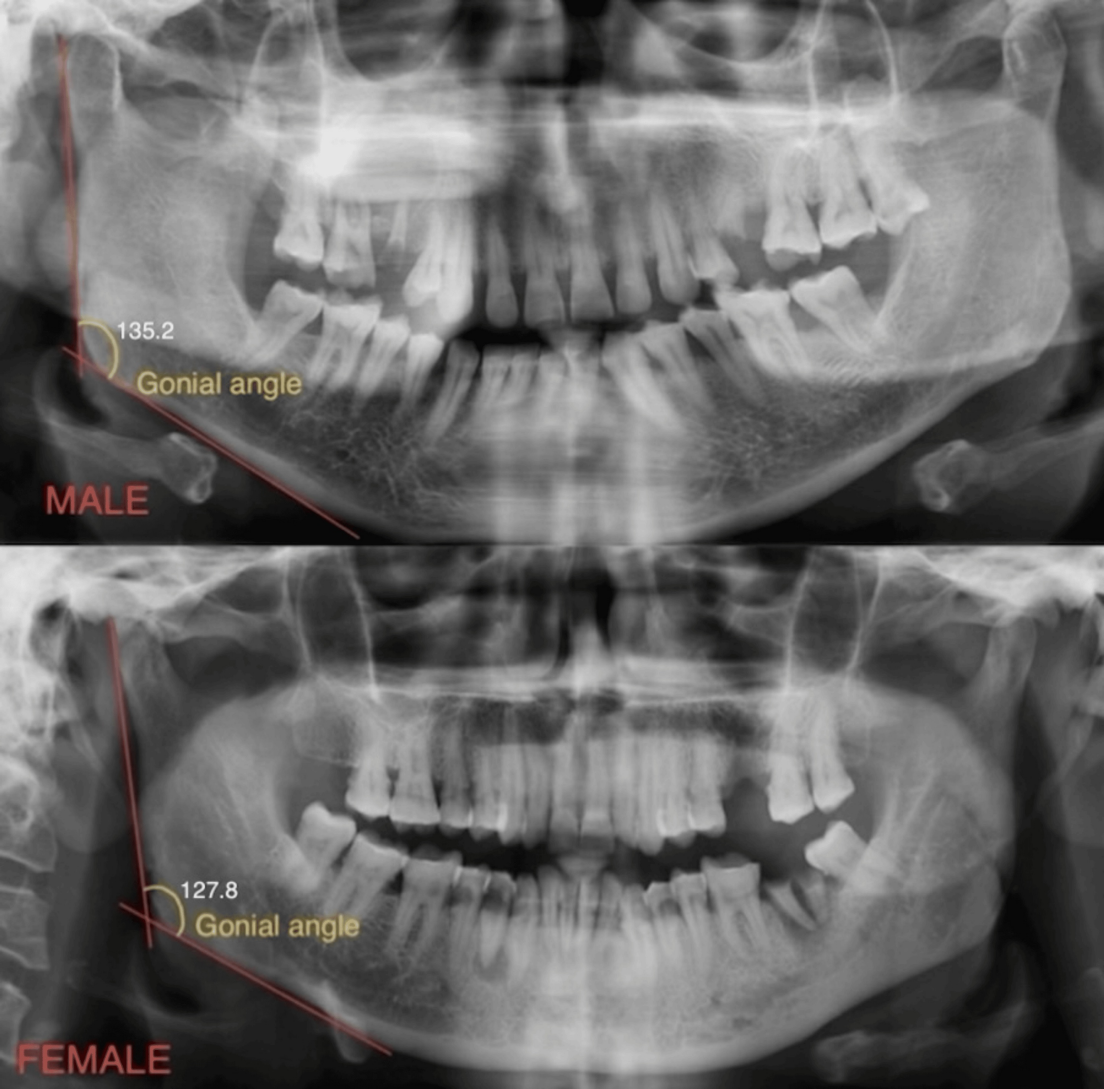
Representation of gonial angle in male and female (51 to 60 years) orthopantomograms, which measures from calculating the tangents to the mandible's lower border and the ramus' posterior border on the orthopantomogram.
